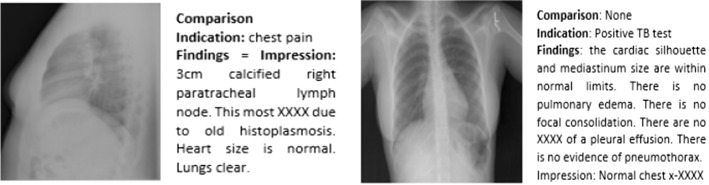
Samples of chest x-ray image-report pairs of two patients and two views (lateral, frontal) from the IU X-Ray dataset (Demner-Fushman et al. 2016)*CheXpert dataset *2 Irvin et al. (2019) is a large dataset of automatically annotated chest X-rays images collected from the Stanford Hospital between October 2002 and July 2017 in both inpatient and outpatient centers. It consists of 224,316 multi-view chest radiographs of 65,240 patients labeled for the presence of 14 common chest radiographic observations as positive, negative, or uncertain. The CheXpert labeler was employed to extract annotations from unstructured radiology reports.*Medical Information Mart for Intensive Care-Chest X-ray MIMIC-CXR dataset*^3^ Johnson et al. (2019) is one of the latest co-released open source datasets that uses the CheXpert labeler for annotation extraction from radiology reports. It includes 371,920 chest X-rays linked to 227,943 reports that have been gathered from the BIDMC between 2011 and 2016. The reports written in English, were de-identified and images pre-processed to remove any information related to the patient (see Fig. 13).
